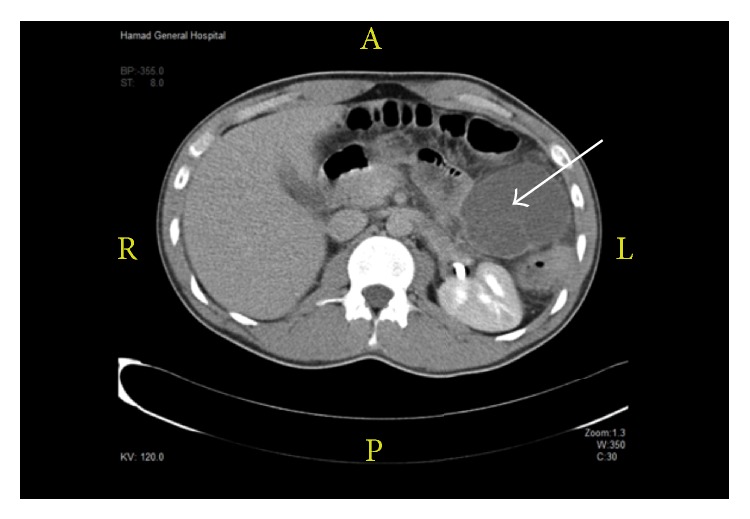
Case 2. Computed tomography scan showing mesenteric cyst (white arrow).
